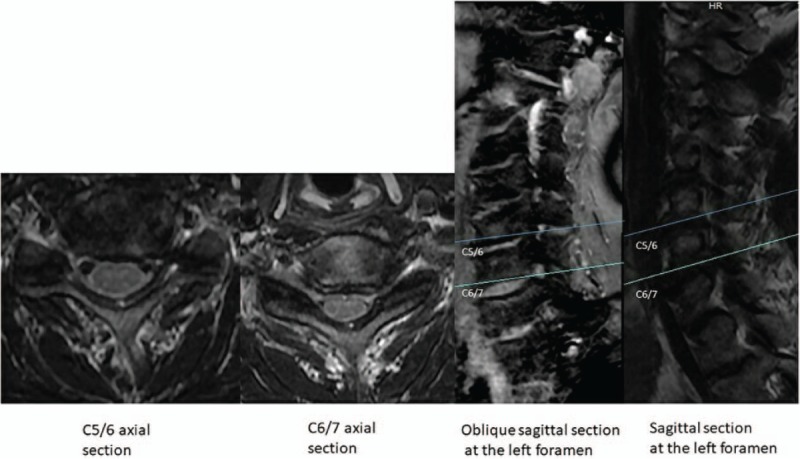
T2-weighted magnetic resonance imaging also demonstrates left C5/6/7 foraminal stenoses. Axial slices are of the C5/6 and C6/7 levels. Oblique sagittal and sagittal slices are of the left foramen.
